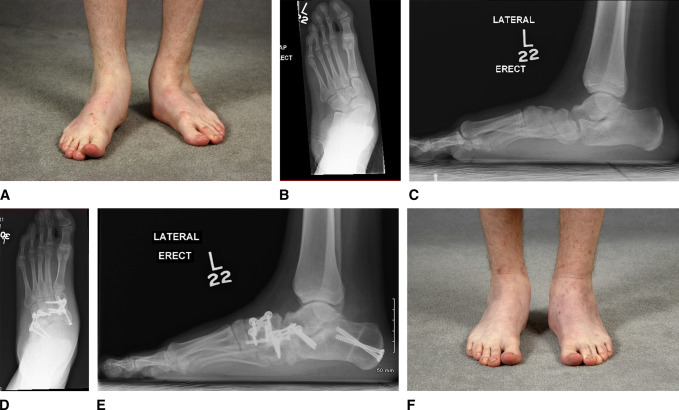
Overcorrection. A–F, Preoperative and postoperative radiographs and photographs of a 14-year-old boy with a history of bilateral congenital clubfoot, who underwent extensive soft-tissue releases at 6 months of age. He presented with pes planovalgus deformity consistent with overcorrection. He was treated with a medial displacement calcaneal osteotomy, calcaneocuboid joint bone-block distraction fusion, midtarsal fusion, advancement of the posterior tibialis tendon, spring ligament reefing, open tendoachilles Z-lengthening, and intramuscular lengthening of peroneus longus and peroneus brevis.
